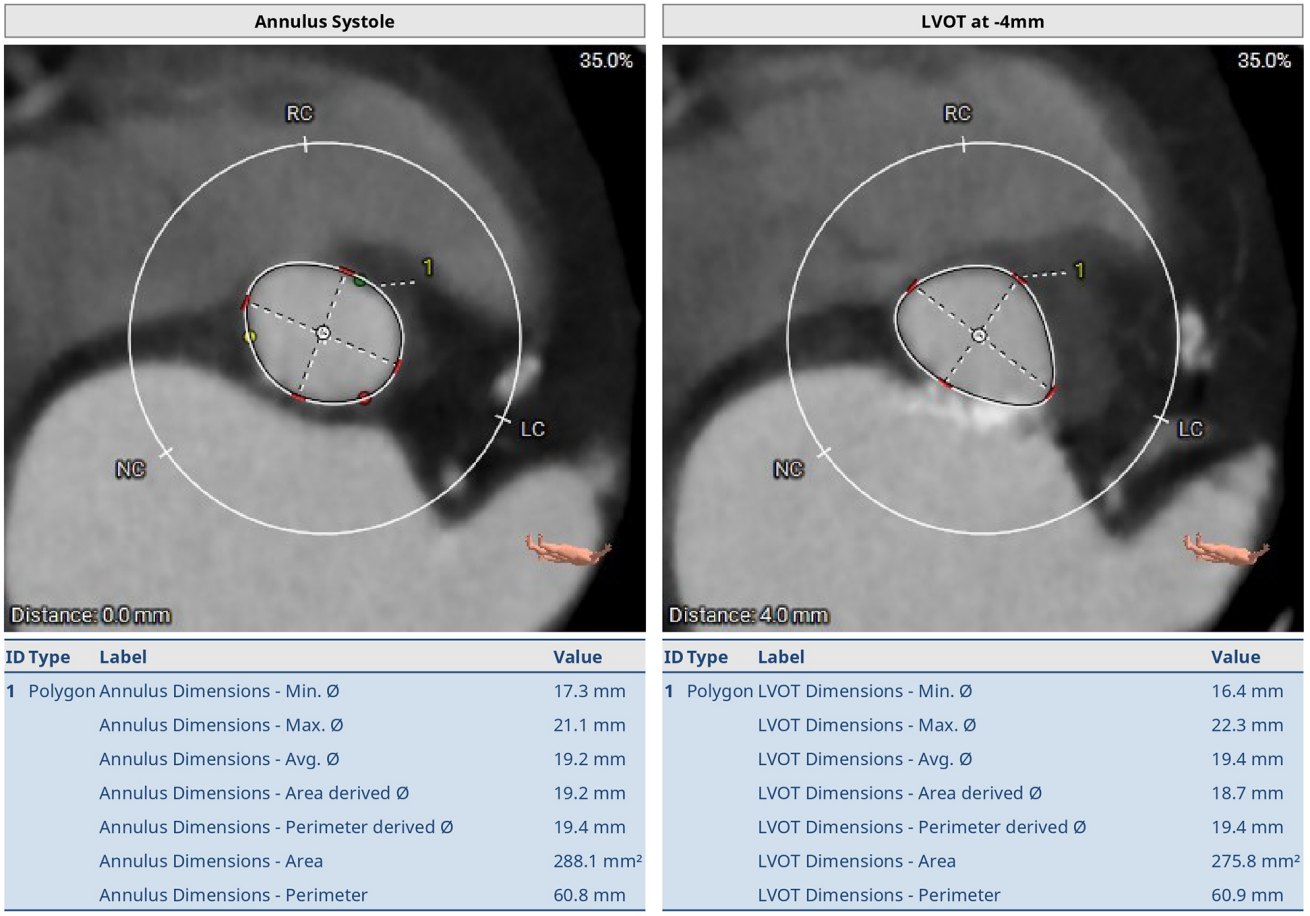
Preoperative thoraco-abdominal CT images showing the annular measurements at systole. The aortic annulus perimeter was 60.8 mm (minor annulus diameter 17.3 mm, major annulus diameter 21.1 mm) and the area was 288.1 mm^2^. The LVOT perimeter was 60.9 mm (minor annulus diameter 16.4 mm, major annulus diameter 22.3 mm) and the area was 275.8 mm^2^.
